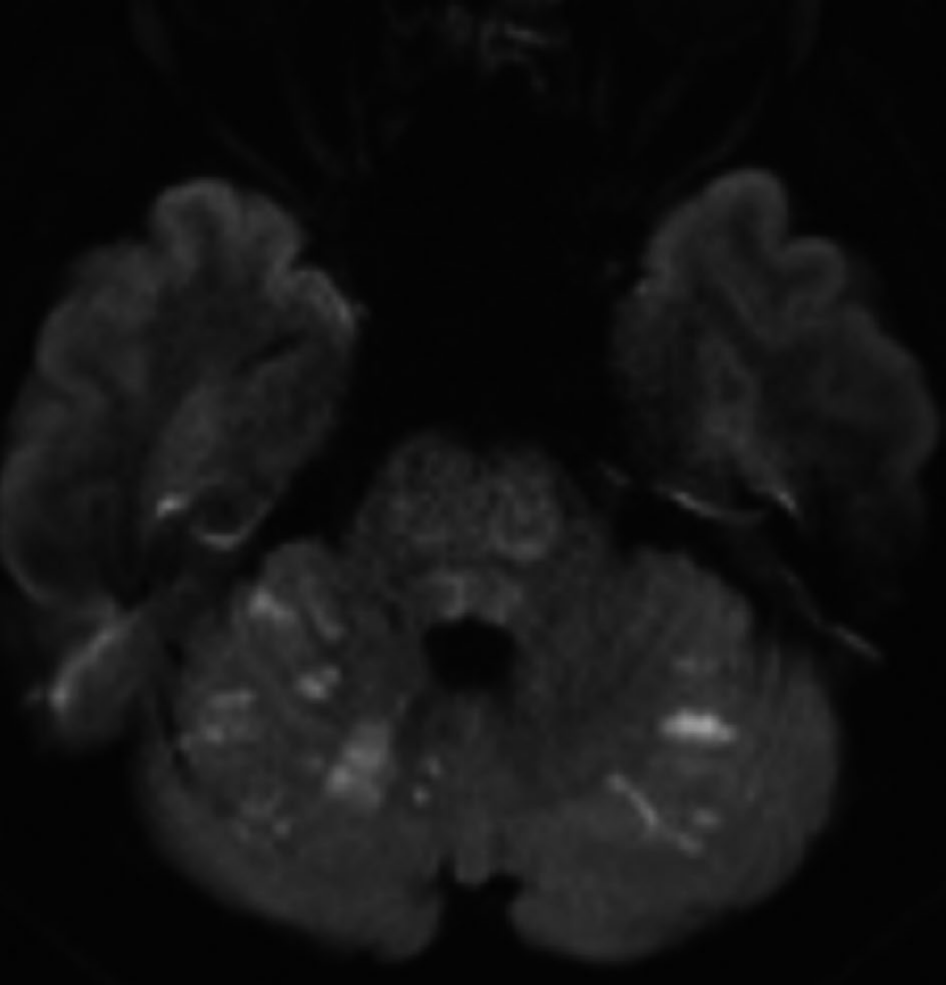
Axial DWI showing restricted diffusion of the pons and bilateral cerebellar hemispheres
